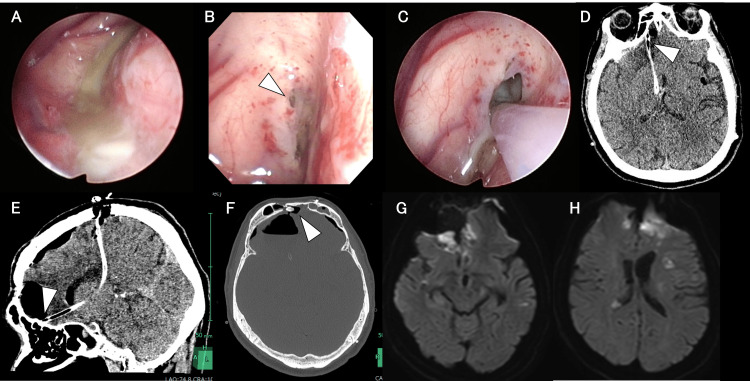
Intraoperative findings (A-C) and postoperative imaging findings (D-H) of endoscopic intraventricular removal in Case 2 A: The right lateral ventricle was filled with abscesses. B: A rupture point between the lateral ventricle and the parenchymal brain abscess was identified (arrowhead). C: A drainage catheter is inserted into the brain parenchymal abscess through the lateral ventricle. D and E: Head CT demonstrating the placement of a drainage catheter into the brain parenchymal abscess cavity through the lateral ventricle (each arrowhead). F: Head CT reveals the presence of air around the opening of the frontal sinus (arrowhead). G and H: Brain MRI-DWI confirmed that the abscesses in the right frontal lobe and right lateral ventricle were mostly removed. CT, computed tomography; MRI, magnetic resonance imaging; DWI, diffusion-weighted imaging
